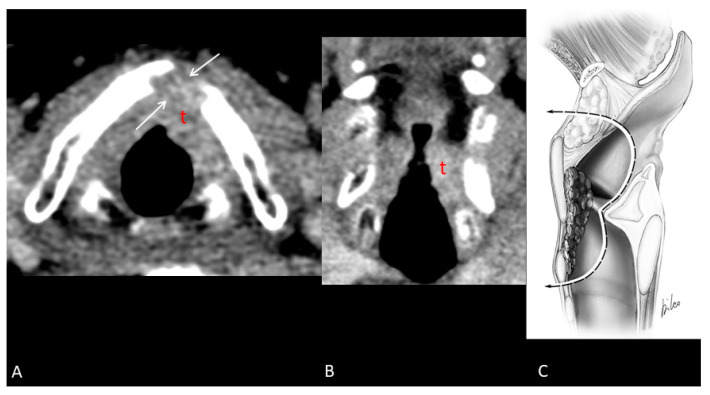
Recurrent glottic SCC after TOLM. Contrast-enhanced CT scan shows neoplastic tissue (t) in the anterior and left sides of glottic level. The lysis (arrows) of the thyroid cartilage is indicative of tumoral invasion at full thickness (A). On the coronal reconstruction, the lesion presents transglottic spread; there is no certain tumoral extension into the subglottic region (B). Sagittal scheme of resection (Type IIIa OPHL) (C) [10].
